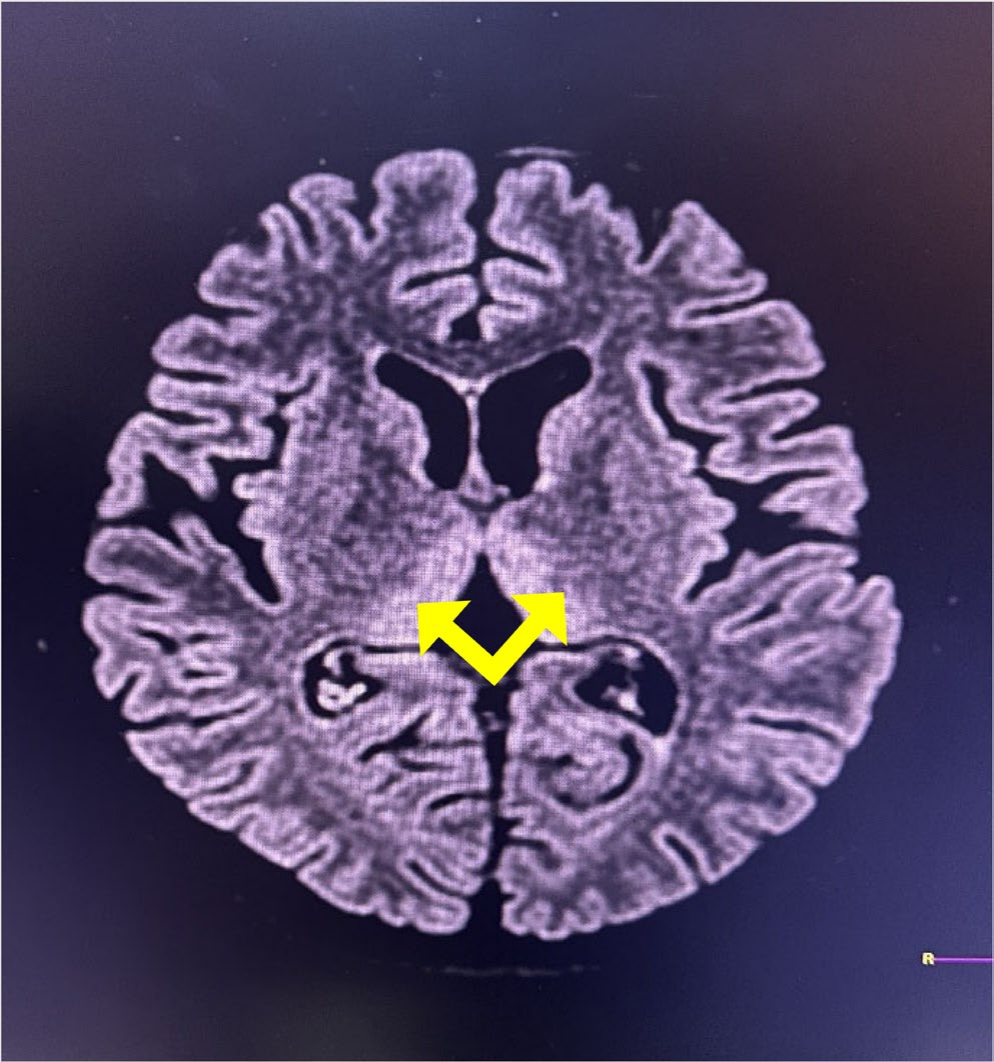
Cerebral MRI T2/FLAIR hyperintensity involving bilateral medial thalami (yellow arrow), suggestive of WE.
